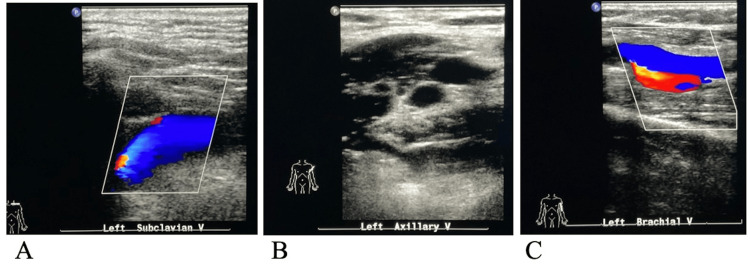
Left upper extremity venous Doppler exam The images do not demonstrate any evidence of deep venous thrombosis in the subclavian (A), axillary (B), or brachial (C) veins
